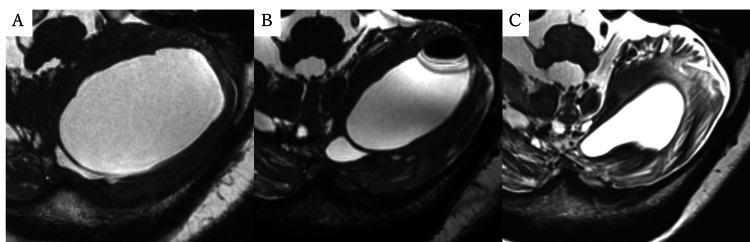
Follow-up heavy T2-weighted MR images demonstrating reduction in cyst size over time (A) At onset of cyst formation (eight months post-op). (B) One month after surgery. (C) Five months after surgery.
